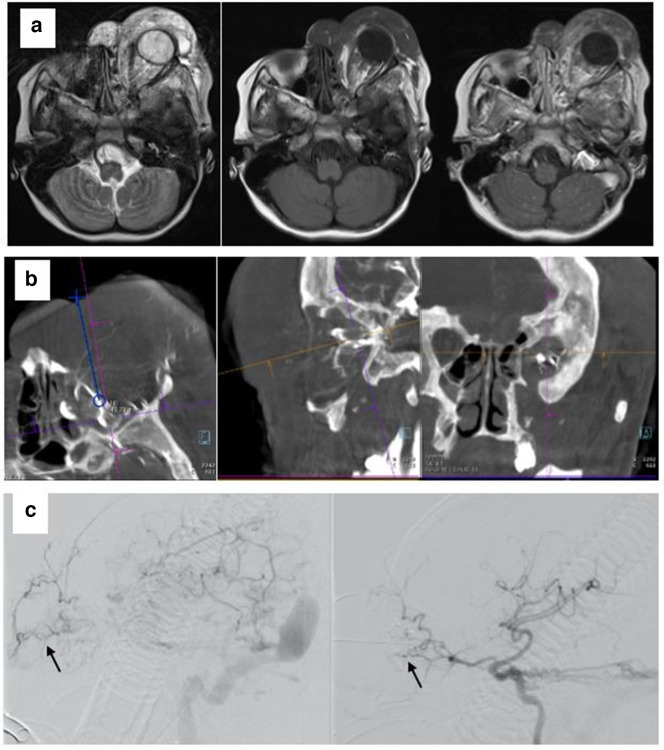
Case 3, Lymphovenous malformation of the frontotemporoorbitozygoma region. (a) Contrast-enhanced MRI revealing a microcystic mass of the left frontotemporo-orbital region with heterogeneous enhancement. (b) CBCT-A in axial, sagittal, and coronal views demonstrating the projection of the virtual needle targeting the left ophthalmic artery. (c) DSA images showing (left) feeding artery, namely the left ophthalmic artery, (right) needle insertion to the target vessel, and occlusion of the feeding artery following glue administration. CBCT-A, cone beam computed tomography angiography; DSA, digital subtraction angiography.
